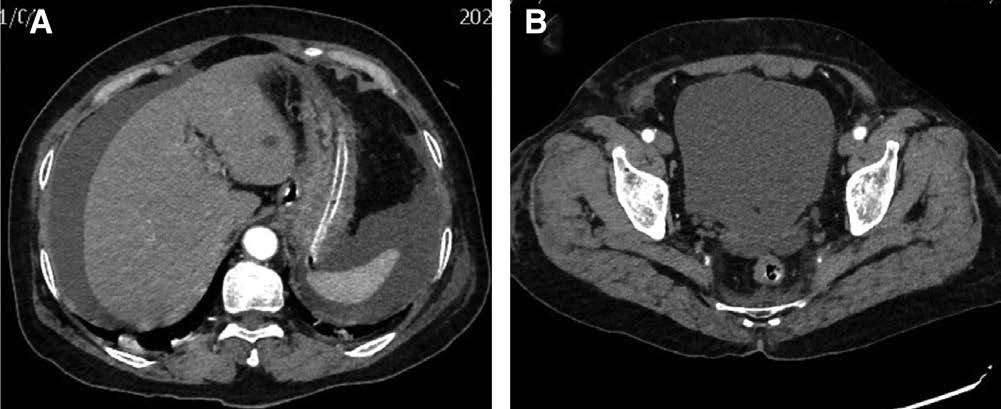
Preoperative abdominal contrast-enhanced CT scan. (A) Substantial volume of free fluid was present in the perihepatic and splenic fossae. (B) The bladder was adequately filled. CT = computed tomography.
